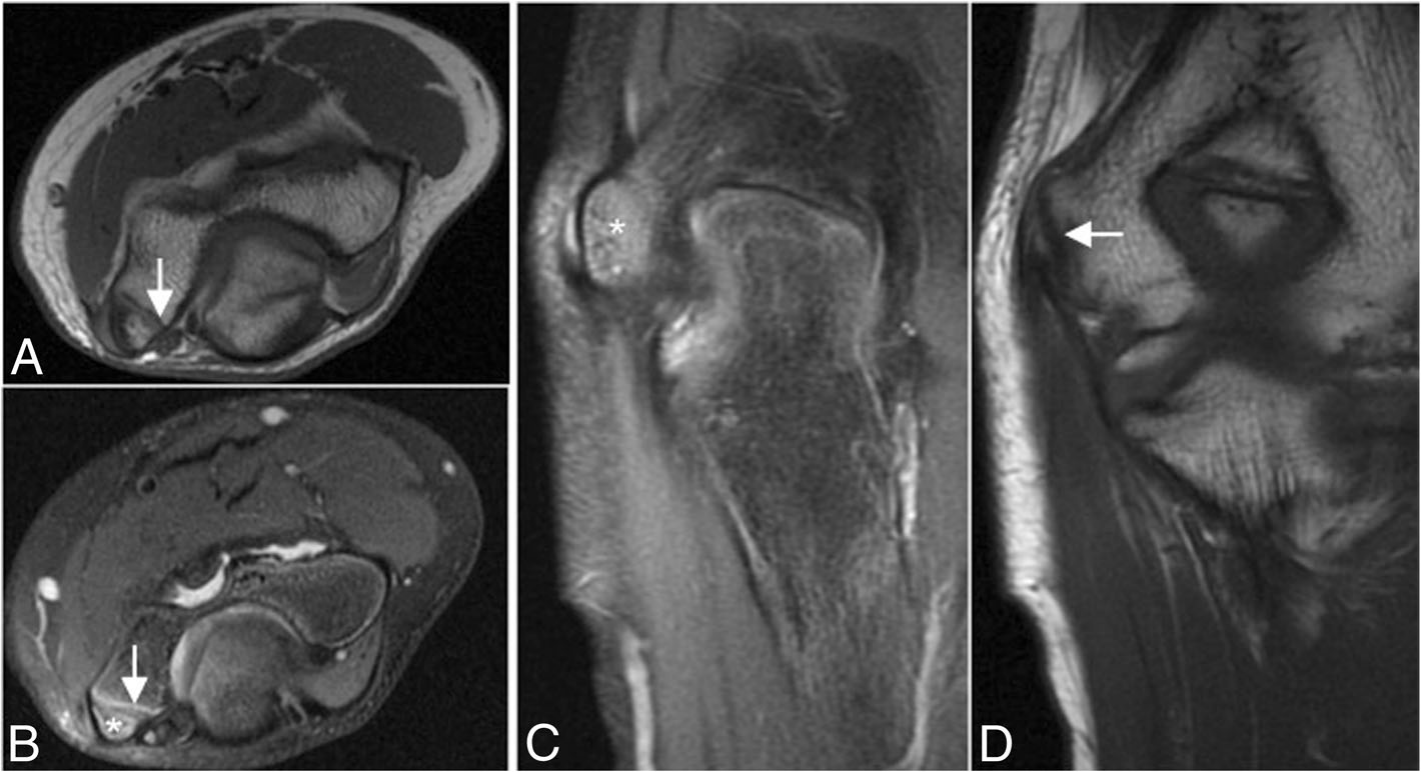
A 12-year-old left-handed baseball pitcher with medial epicondyle pain. Axial T1-weighted MRI (a), axial FS PD-weighted MRI (b), coronal FS PD-weighted MRI (c), and coronal T1-weighted MRI (d) showing a widening of the medial epicondylar physis (white arrows) and bone marrow edema (white asterisks)
